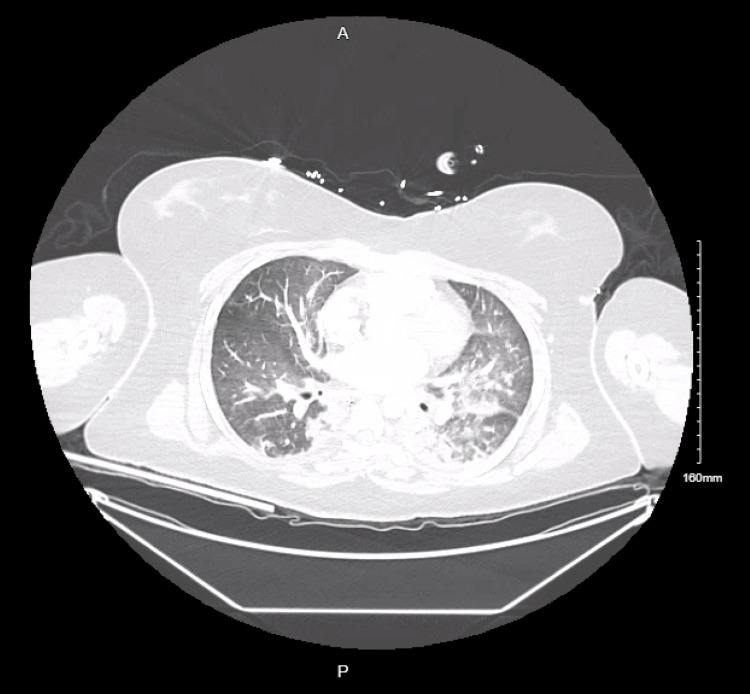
CT chest (axial view) Bilateral, posterior-predominant airspace disease is seen with multifocal ground-glass opacities and superimposed consolidation, consistent with a mixed alveolar–interstitial pattern. Findings are symmetric with relative anterior sparing. No nodules, cavitation, pneumothorax, or large pleural effusions are identified, and the central airways are patent.
